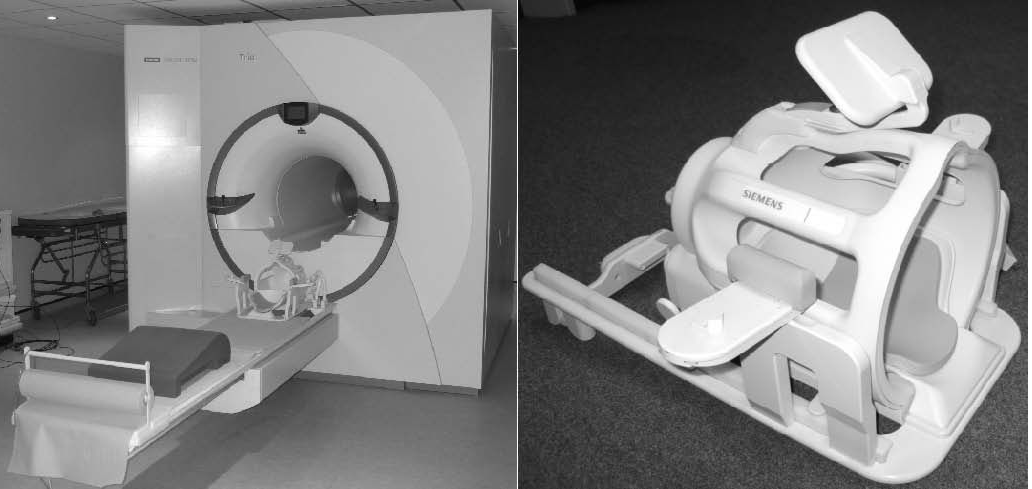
MRI equipment. Siemens Trio 3 Tesla MRI scanner (left) and the used array head coil with attached mirror to enable visual stimulus presentation (right). During scanning two additional foam cushions, one on each side, were placed between the fasteners and the participant's head to further shield the noise and reduce head motion.
